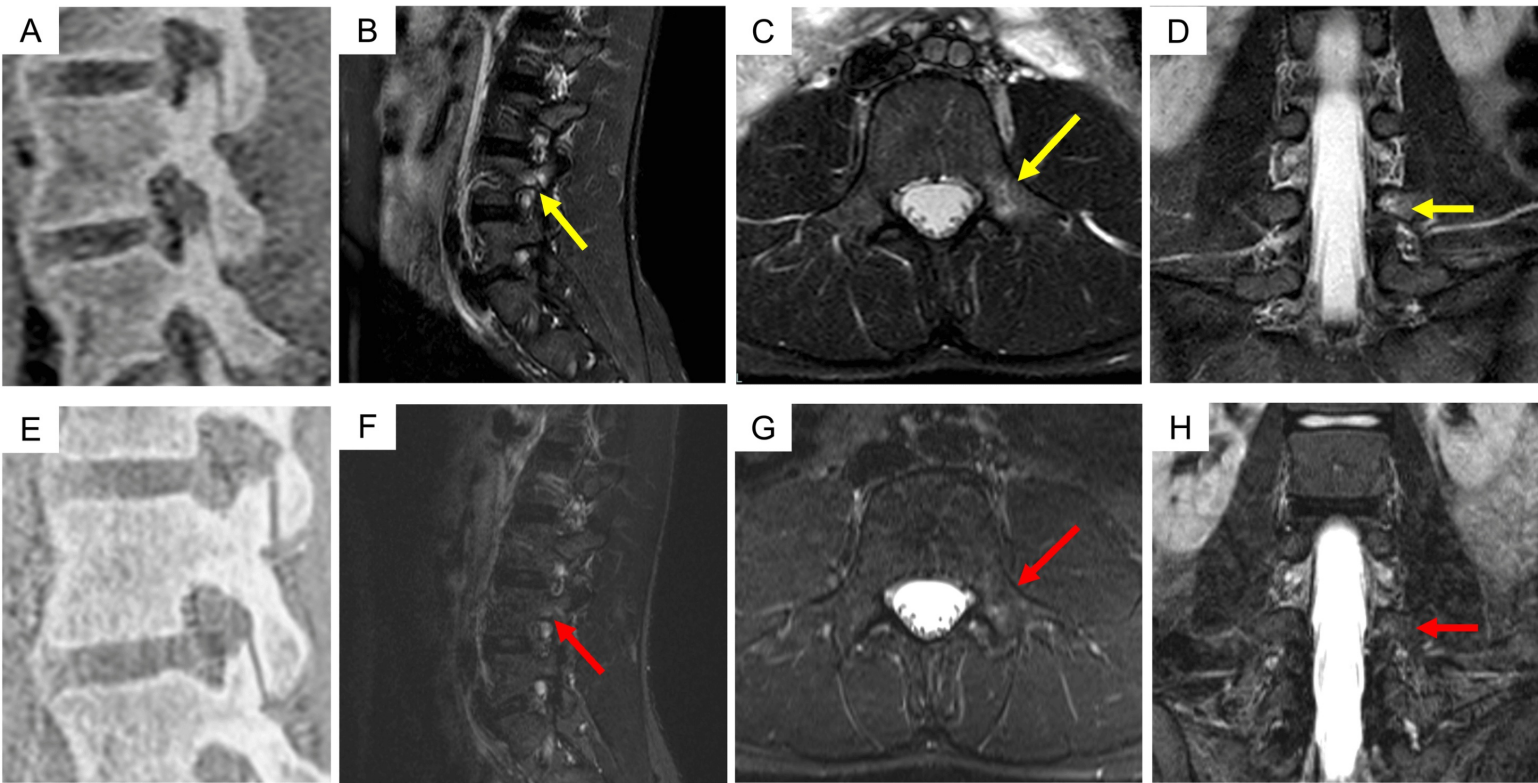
MRI images of Case 5 showing a lesion at the left L4 pars interarticularis. MRI scans obtained at the initial visit (A–D) and one month later (E–H) are presented. No fracture lines were visible on the initial MRI images; however, BME was observed (yellow arrows). At the one-month follow-up, the BME had resolved (red arrows). MRI, magnetic resonance imaging; BME, bone marrow edema; A, B, E, and F, sagittal view through the right L4 pedicle; C and G, axial view through L4; D and H, coronal view through the left L4 pedicle.
